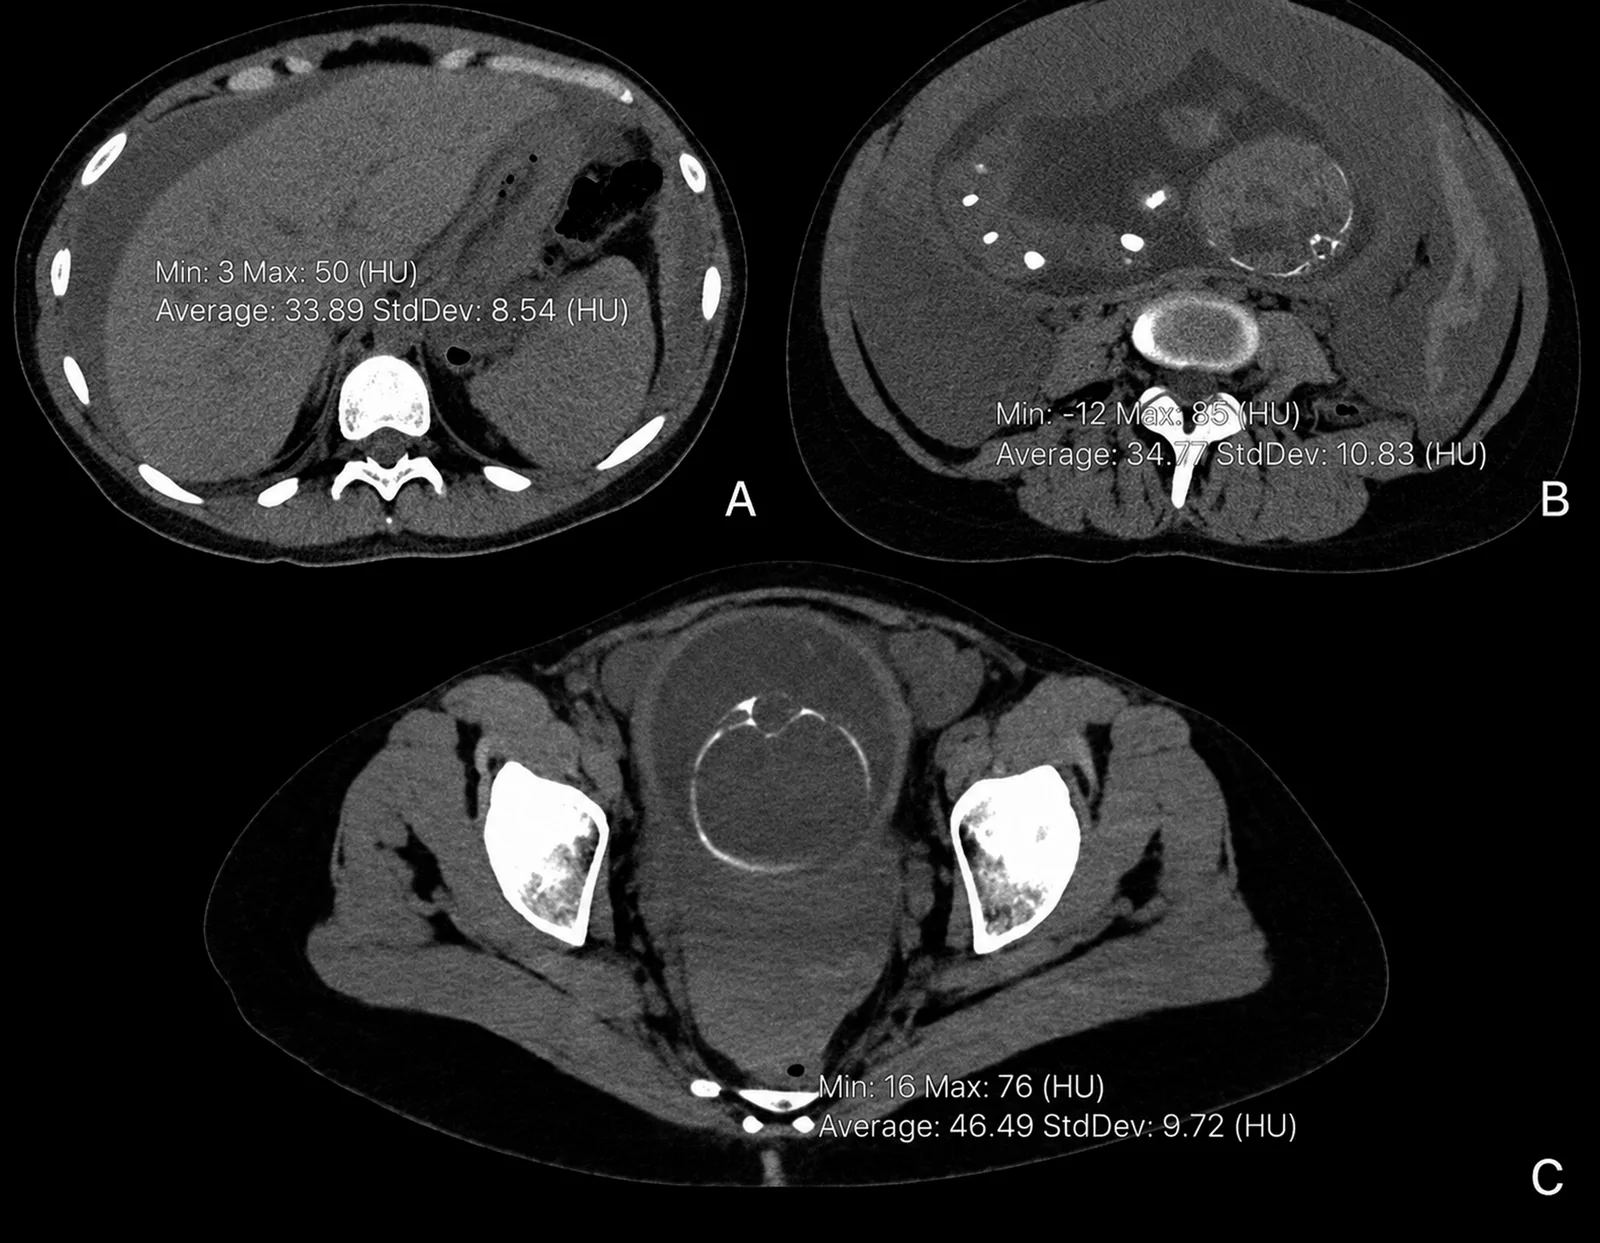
Axial non-contrast CT images of the abdomen and pelvis demonstrating hemoperitoneum at three levels: perihepatic region measuring 25.89 ± 8.54 HU (
